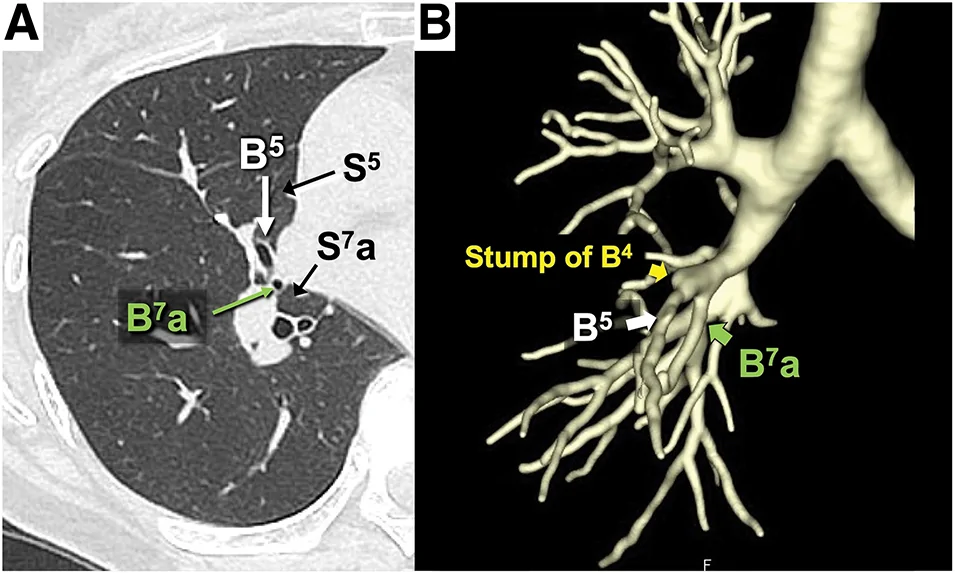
Postoperative CT and 3D reconstruction images. (A) S^5^ and S^7^a were preserved with the intact bronchi. (B) B^5^ and B^7^a were intactly preserved.
